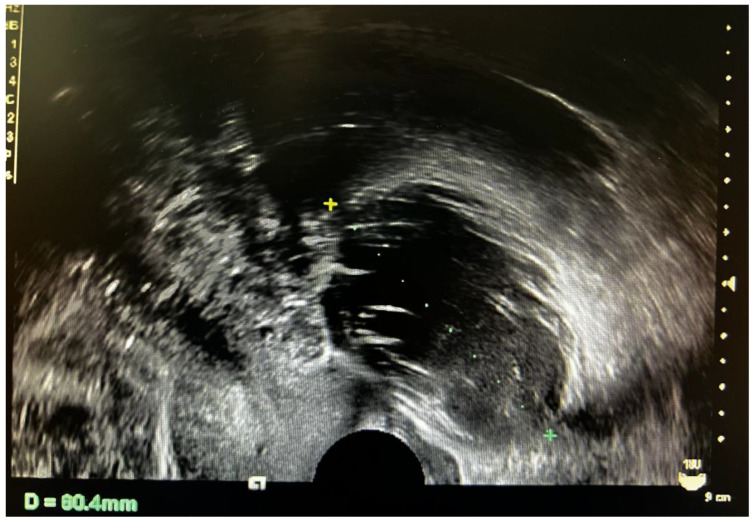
Ultrasound revealed a mixed echogenic zone on the left side of the uterus, 55 × 60 × 46 mm, with a poorly defined boundary, medium hyperechogenic mass in the inner part, 21 × 12 × 18 mm, and sparse color blood flow on the surface and inside.
